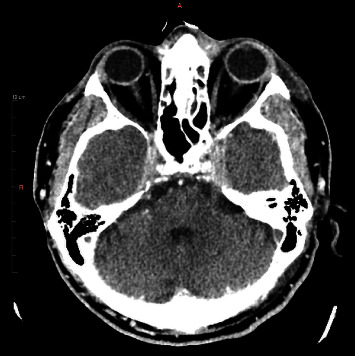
Contrast-enhanced brain CT showing thrombosis of multiple venous cerebral sinuses. The paranasal sinuses, as well as the middle ear and mastoid cavities, are free from fluid collection.
